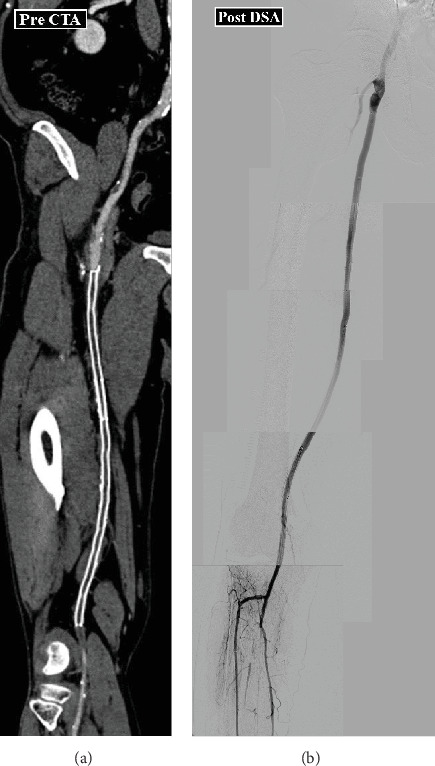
Pre- and postprocedural results. (a) Preprocedural computed tomography angiography. (b) Postprocedural distal subtraction angiography.
